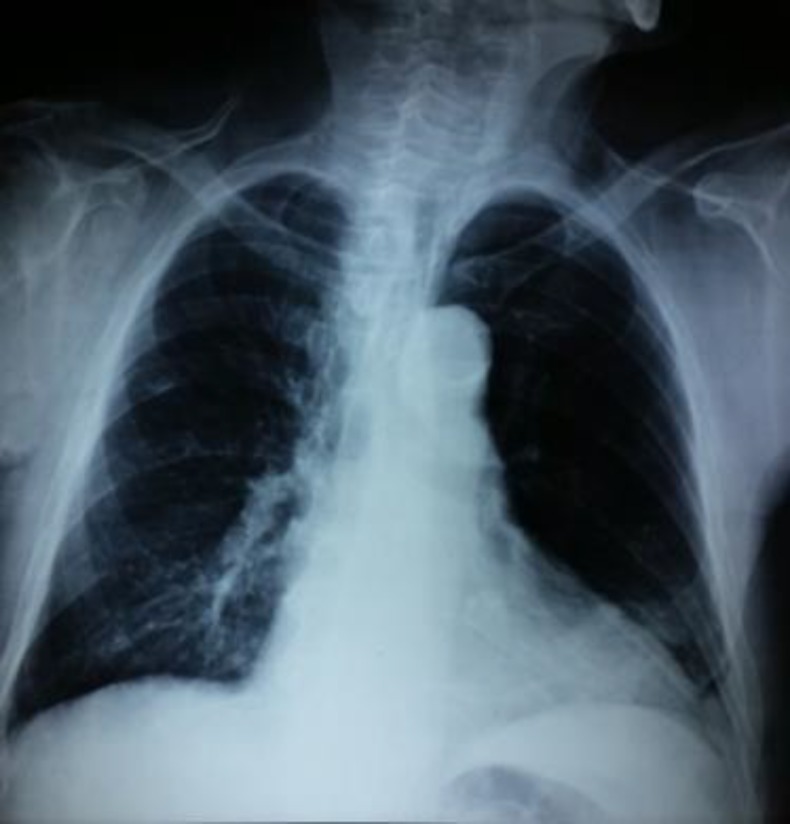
Patients Chest x-ray demonstrating a retrocardiac left lower lobe consolidation
